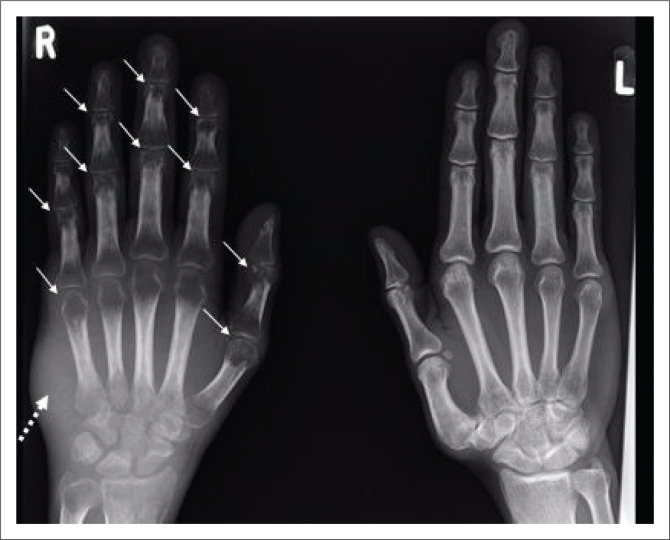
A 40-year-old human immunodeficiency virus-negative male presented with a 2-month history of unilateral right hand swelling. A conventional radiograph of the hands demonstrates diffuse periarticular osteopenia (solid white arrows) and right-sided soft tissue swelling (white arrow with dotted line). No erosions or joint space narrowing were observed. Synovial biopsy confirmed tuberculous tenosynovitis.
